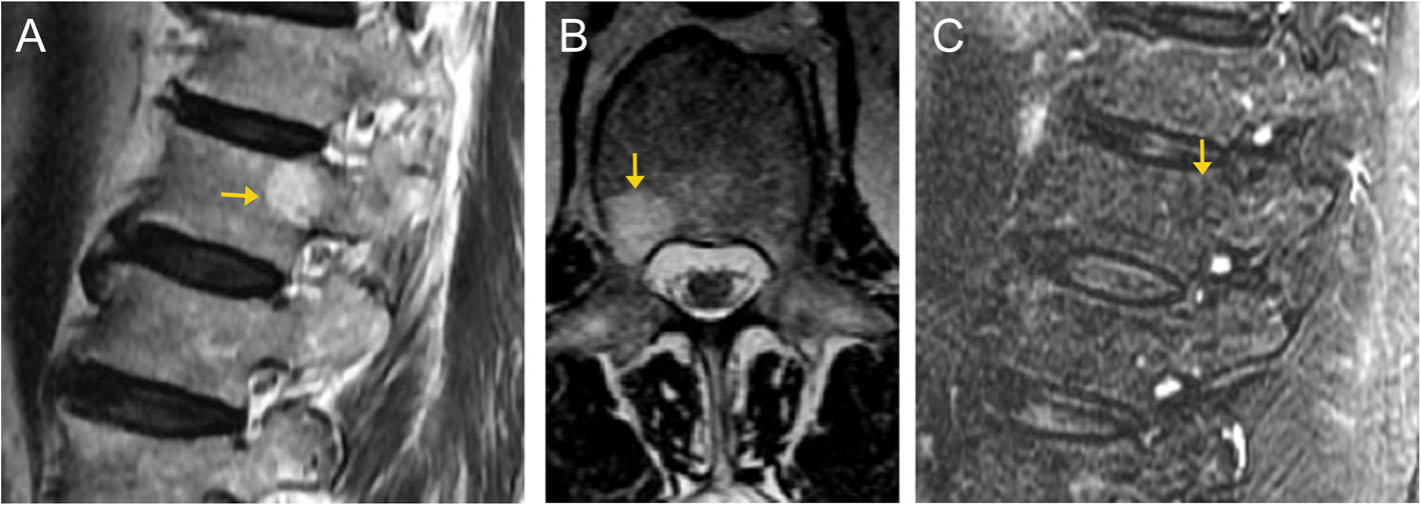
Vertebral venous vascular malformation. Sagittal (a) and axial (b) T2-weighted MR images demonstrate a well-defined lesion in the right posterolateral aspect of T12 (arrows). The lesion is hyperintense relative to the surrounding normal marrow, and demonstrates suppression (arrow) on the T2 fat-suppressed sequence (c). This was an incidental finding and diagnosed radiographically as a vascular malformation. Given the lack of expansion into the spinal canal or compression of the cord, unlike the prior case, this is considered a “do not touch” lesion
